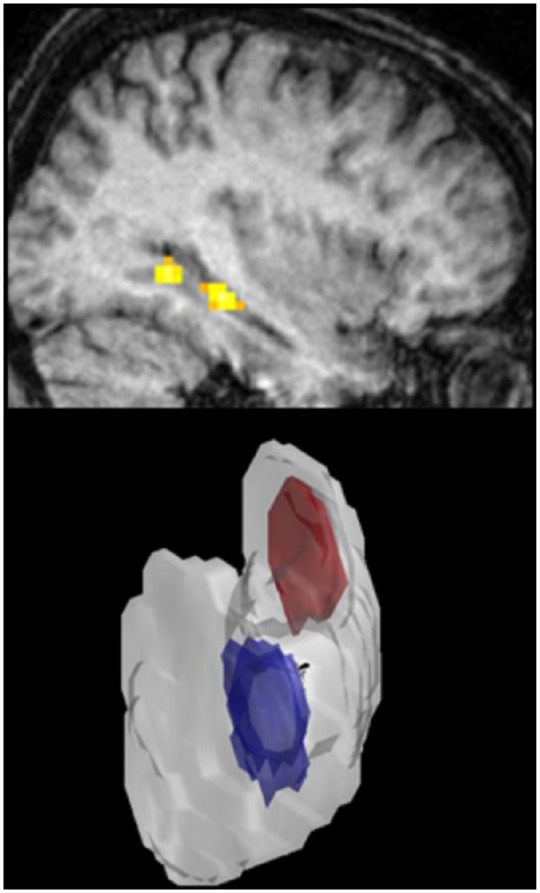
Intrahippocampus considerations. Top panel shows activation of the residual tissue in the right hippocampus of a patient with bilateral hippocampal damage when he constructed scenes in his imagination (from Mullally and others 2012a). Lower panel shows a three-dimensional rendering of a healthy hippocampus from an example participant in the functional magnetic resonance imaging study of Bonnici and others (2012). The blue area indicates where information about recent memories was detectable (anterior hippocampus) and the red area shows where more information was detectable about remote memories (posterior hippocampus).
